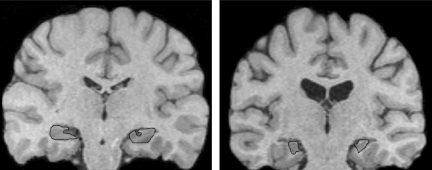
Selected coronal slices of a FLASH MRI scan in an age- and sex-matched healthy control (left), and in HC (right). The hippocampi are outlined in black, and are markedly decreased in volume for HC.
